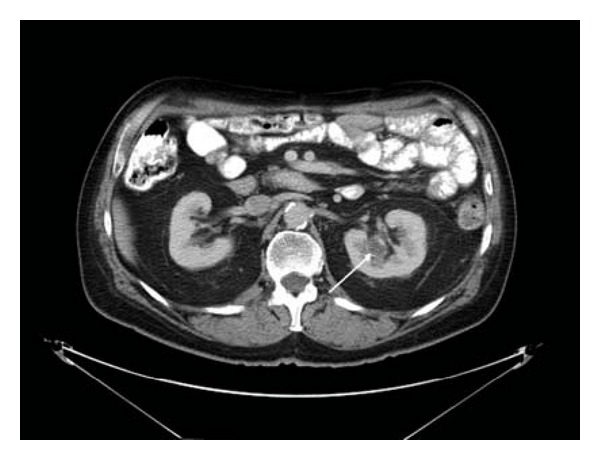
CT scan of the abdomen showing a 2.2 cm solid lesion in the midpole of the left kidney near the pelvis (white arrow).
